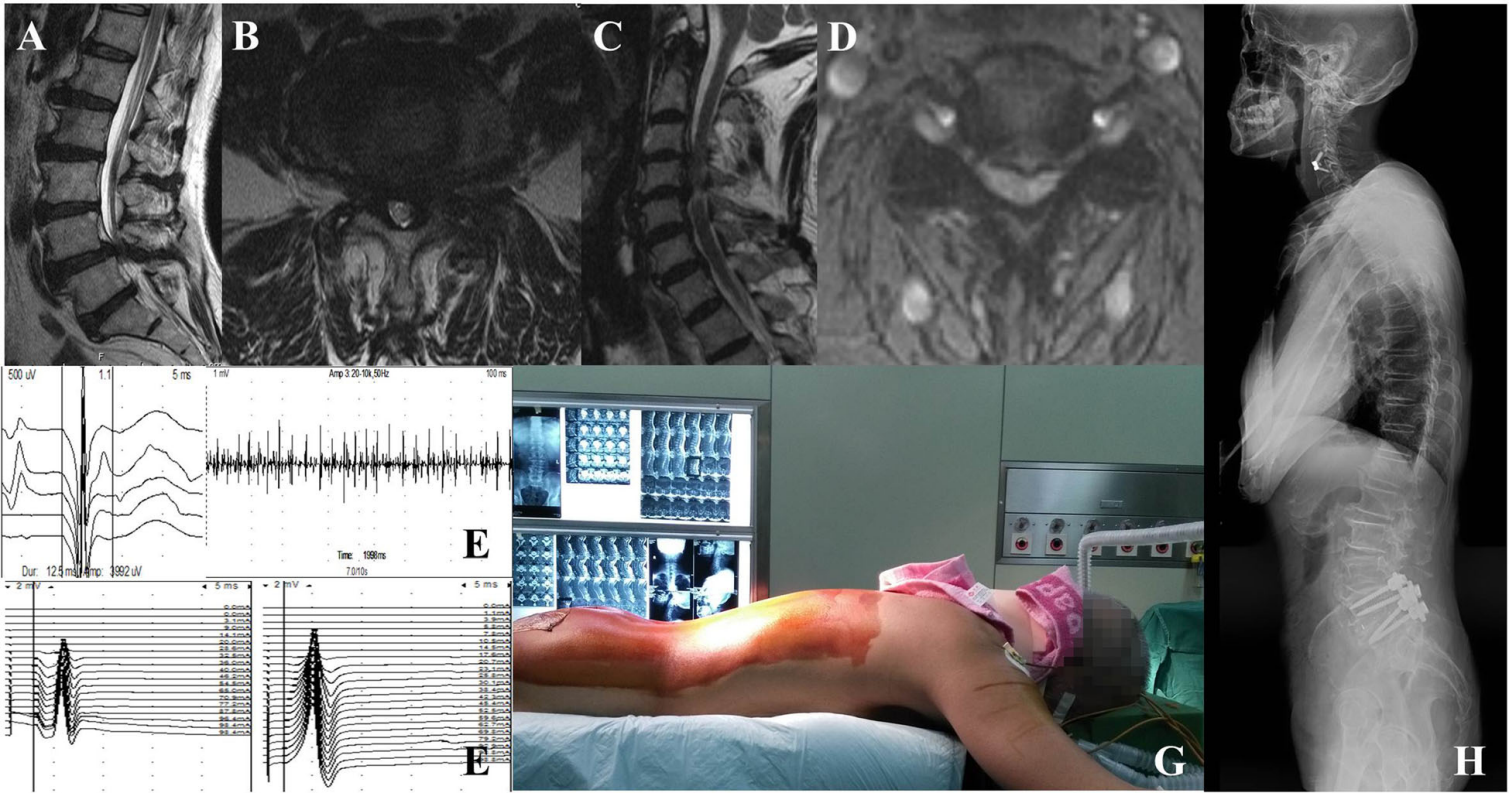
A 68-year-old man had numbness and acratia in the upper extremities and neurogenic claudication. Preoperative magnetic resonance imaging (MRI) showed listhesis at L4 and grade 3 stenosis at L4–5 (a, b) and disc herniation at C4–5 associated with grade 3 compression (c, d). The biceps brachii showed motor unit potentials (MUP) with a prolonged duration, increased amplitude, and a simple-mixed phase in the recruitment order (e). A bilateral tibial nerve H-reflex was not elicited (f). The simultaneous operation was performed using ACDF first and PLIF next, with protection of the neck collar (g). Postoperative x-ray of the whole spine showed internal fixators in both the cervical and lumber spine (h)
